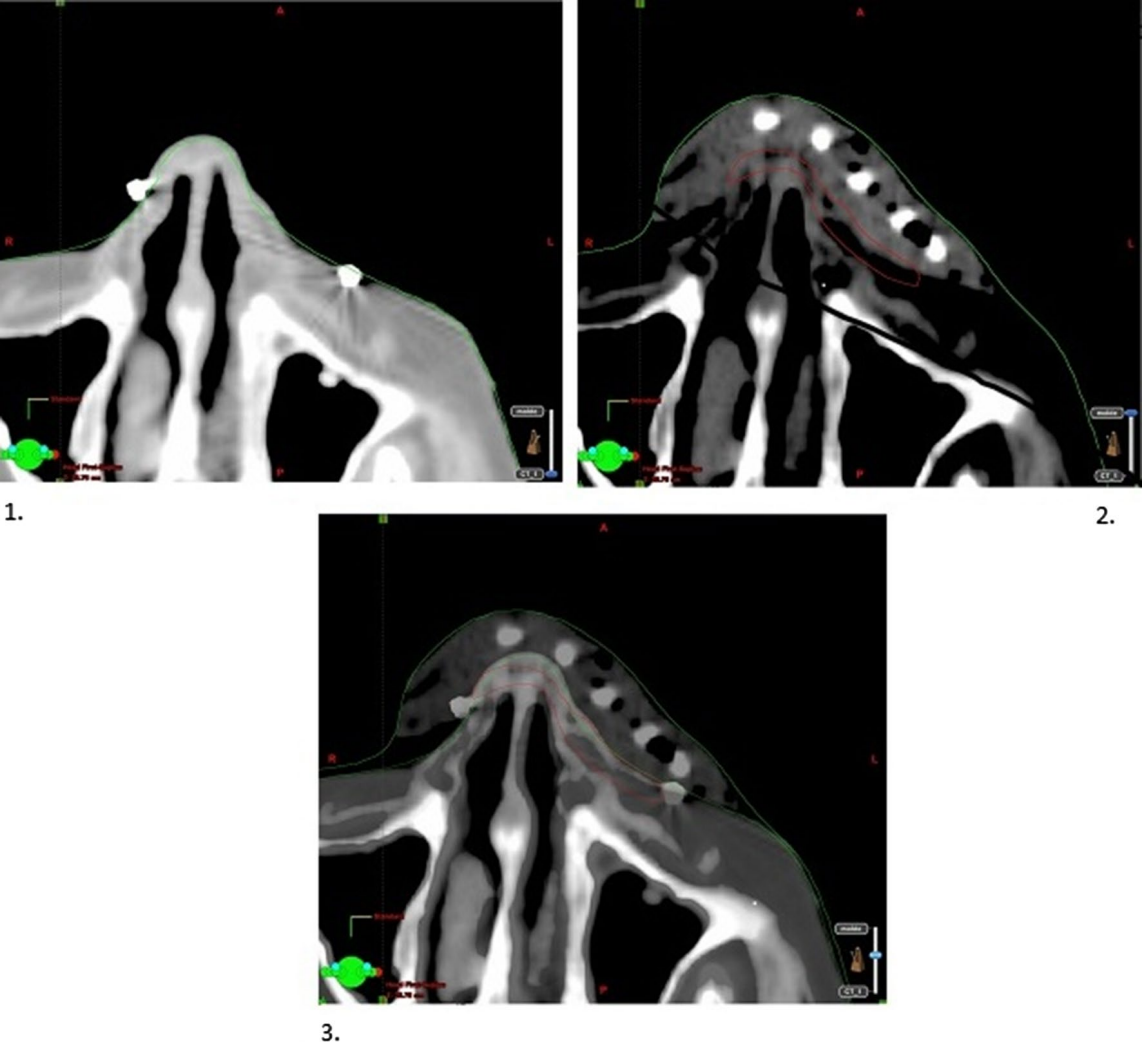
1—Computed tomography (CT) image with radiopaque marker in place. 2—CT image with customized mold in place. 3—Fusion of images for treatment planning
